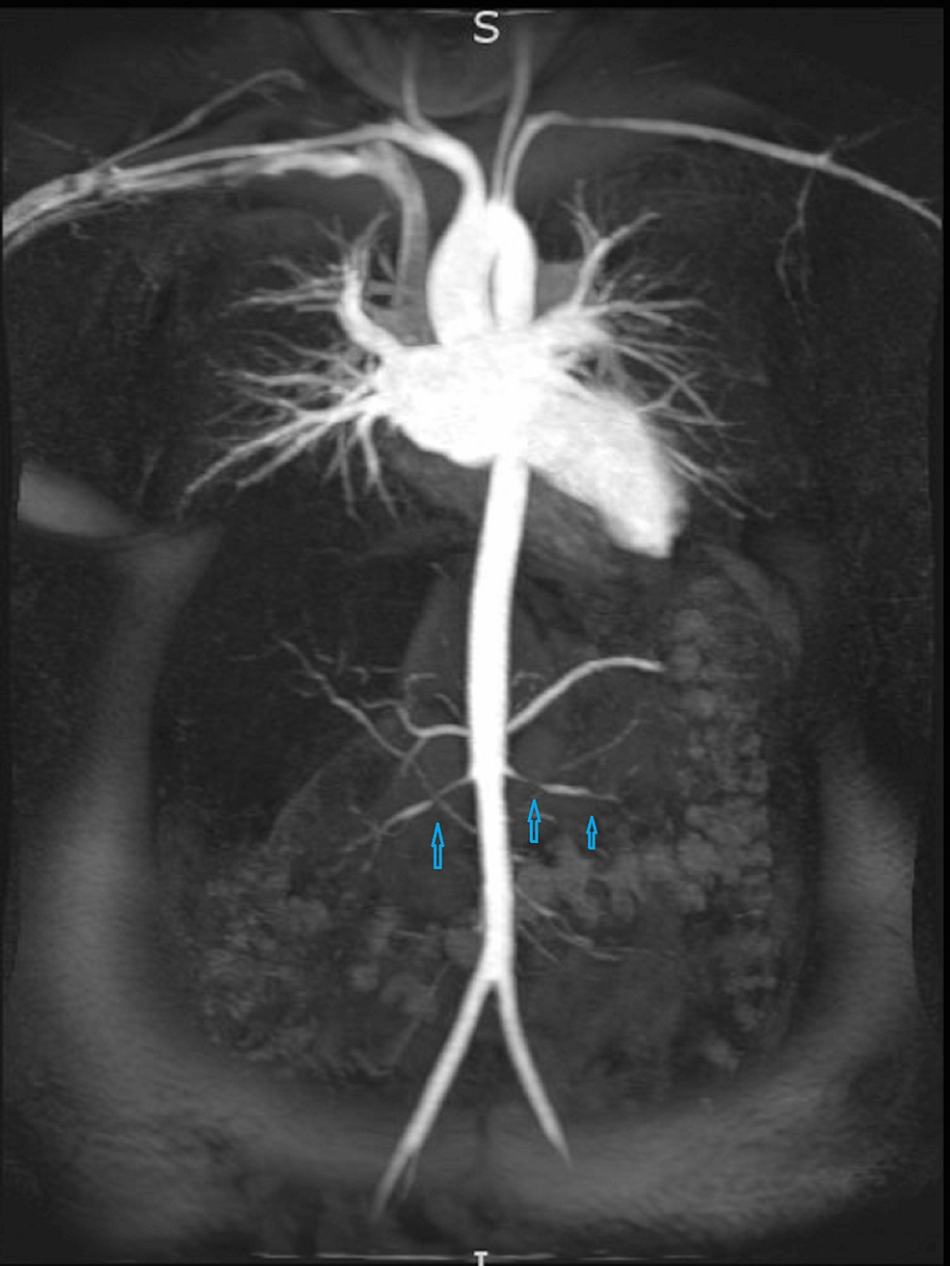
MRI showing FMD of renal arteries as shown by arrows. FMD, fibromuscular dysplasia
